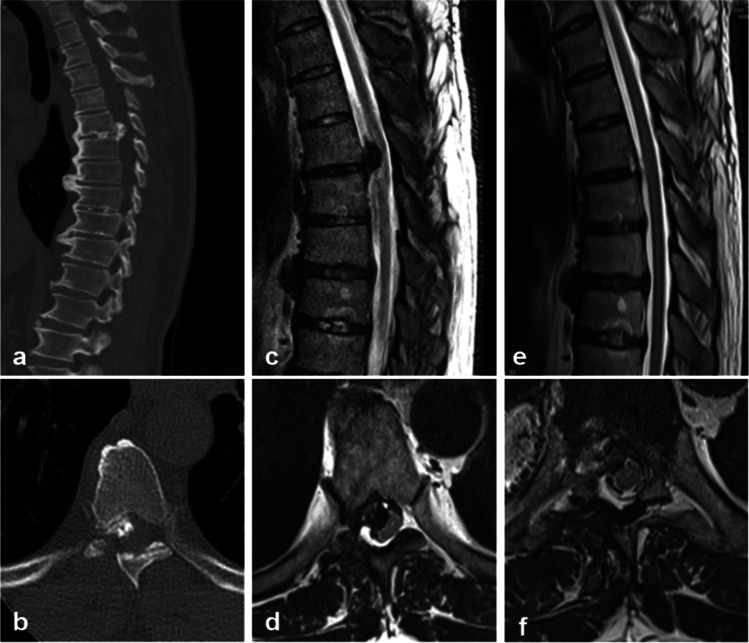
Preoperative (a–d) and postoperative (e–f) MRI of patient No. 5 with a mediolateral left-sided, partially calcified disc herniation at level Th 7/8 causing relevant spinal cord compression, with spinal cord and radicular compression completely removed in (e) and (f)
